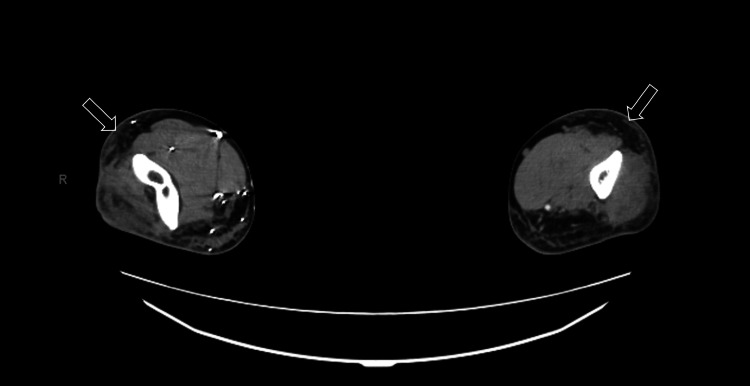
CT angiogram with contrast of upper bilateral extremities
